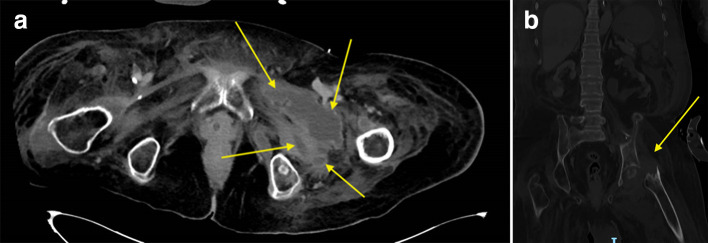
(a) CT axial image at the level of the hips demonstrating multiple inoculated abscesses around the left hip joint area (yellow arrows). (b) CT image in the coronal plane showing complete destruction of the left hip joint (yellow arrow), visualized under Bone window.
